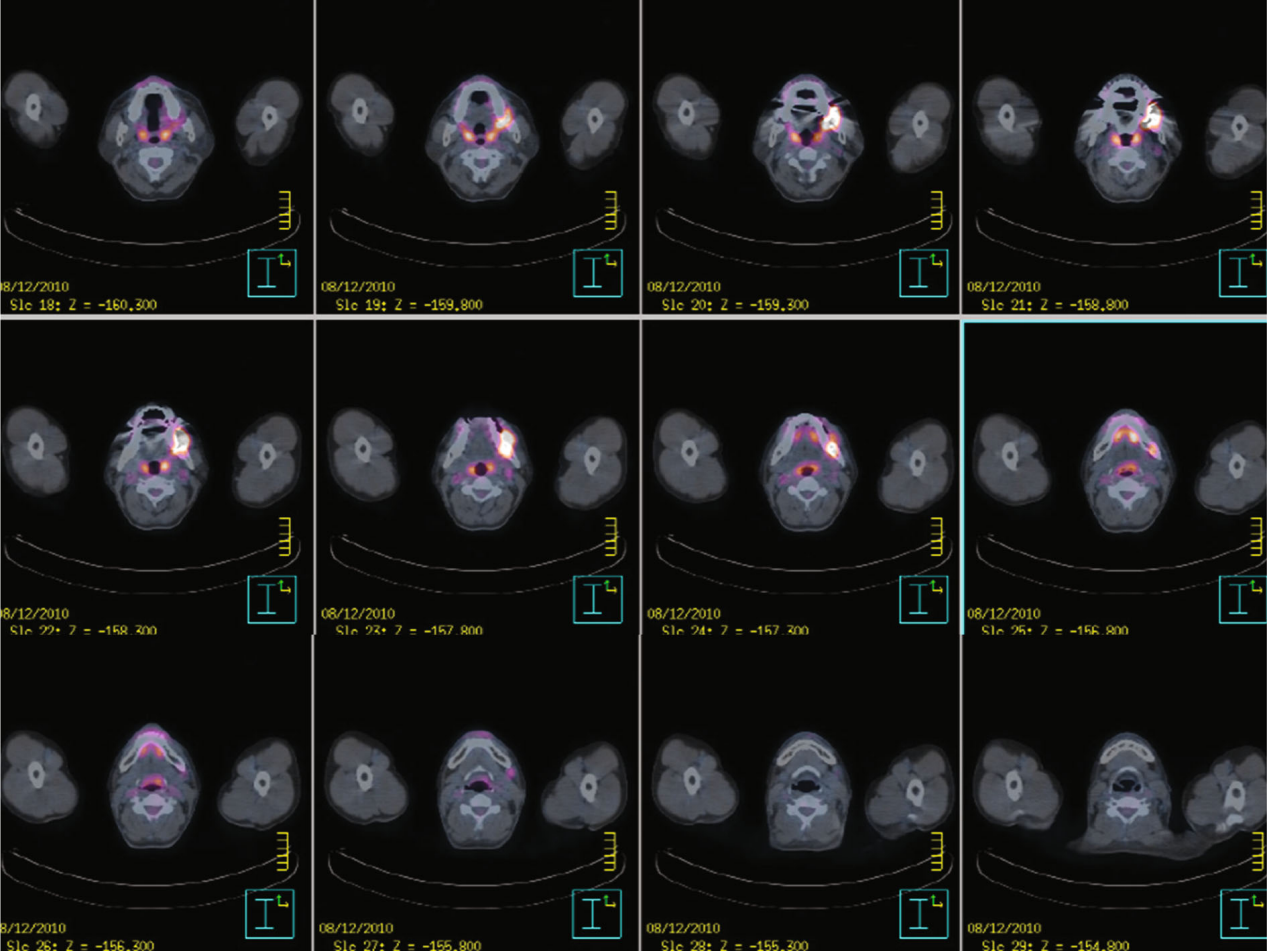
A positron emission tomography-computed tomography scan shows a focal hypermetabolic lesion in the left part of the oral cavity and multiple mild hypermetabolic lesions in the left cervical level Ib, II, and V.
